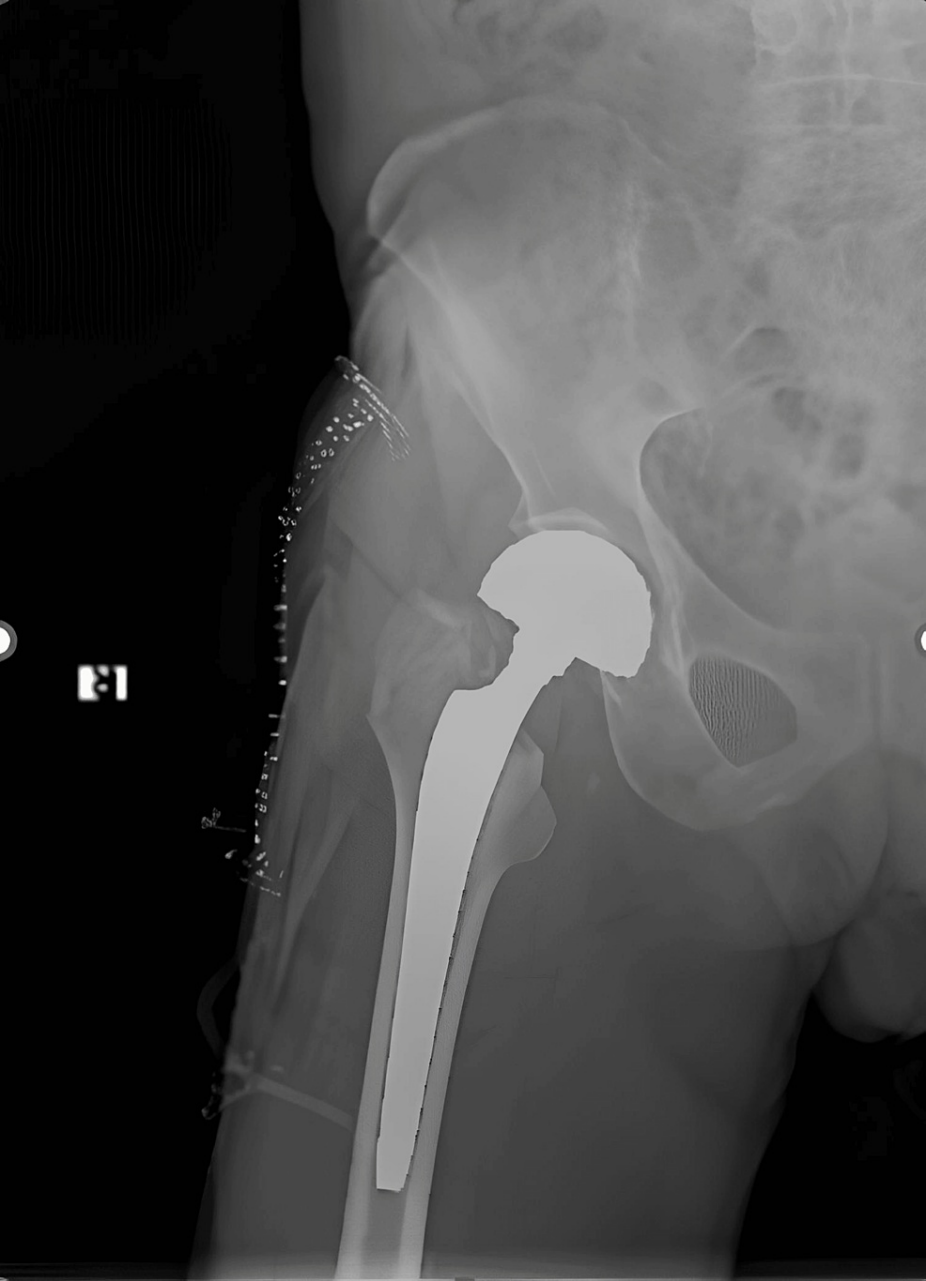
Postoperative X-ray image of femoral neck fracture treated with hemiarthroplasty
